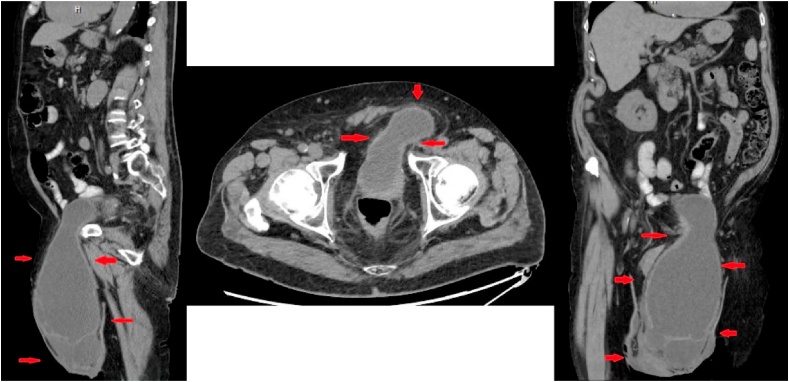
CT abdomen and pelvis without contrast revealing a herniation of 80% of the bladder through the left inguinal canal into the left scrotal sac.
